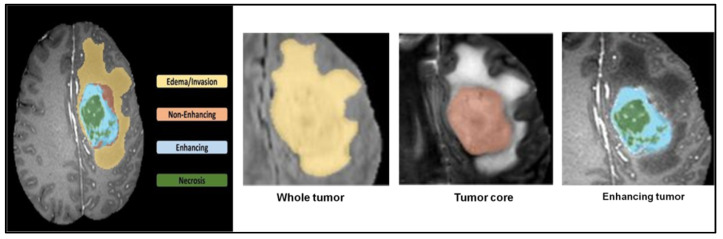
3D MRI brain tumor semantic segmentation.
